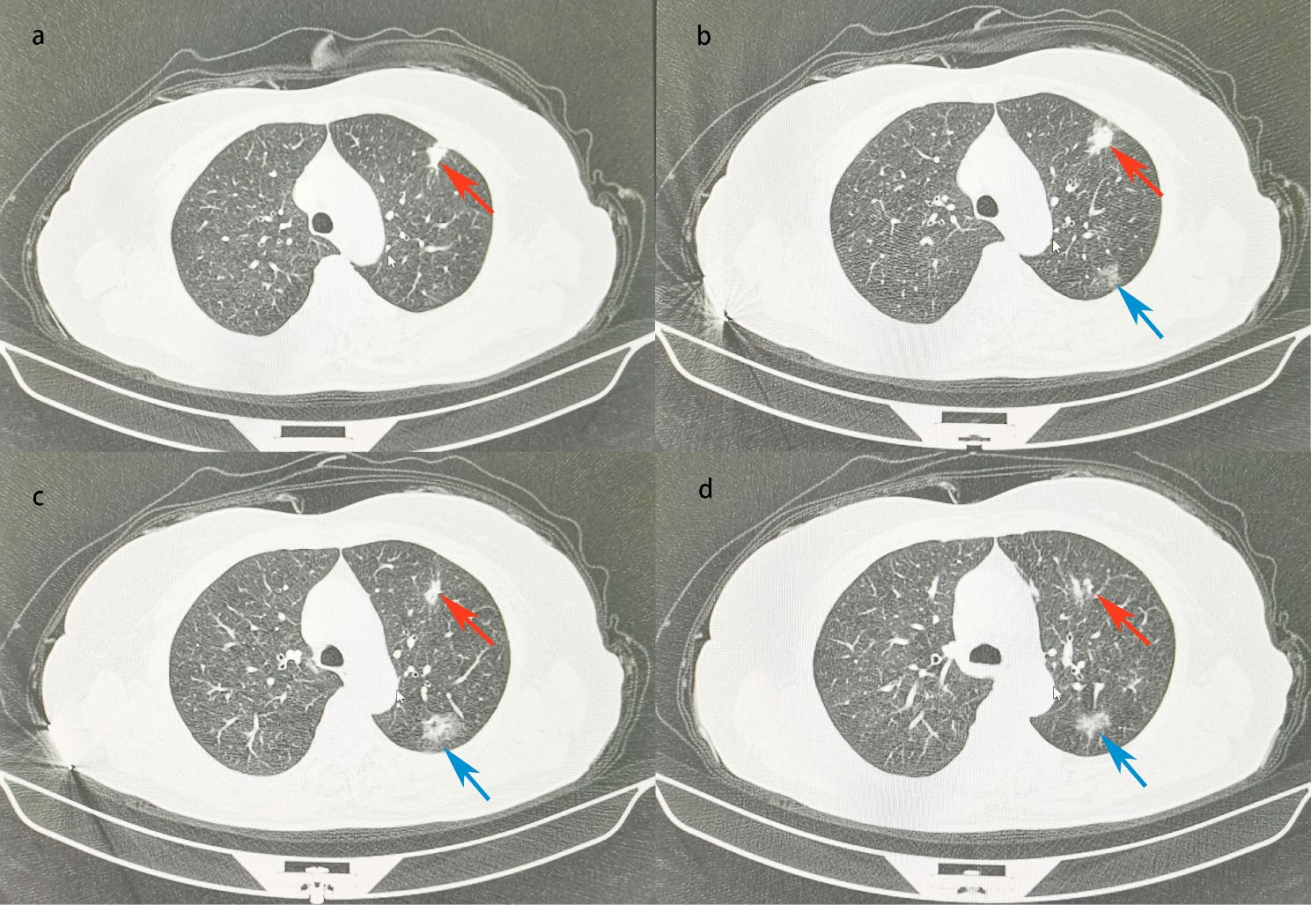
Chest CT showed an irregular solid nodule with a size of about 1.7 cm*1.1 cm in the S3 segment of the left lung (red arrow), and a mixed ground-glass nodule with a size of about 2.6 cm*1.8 cm in the S1 + 2 segment of the left lung (blue arrow)
